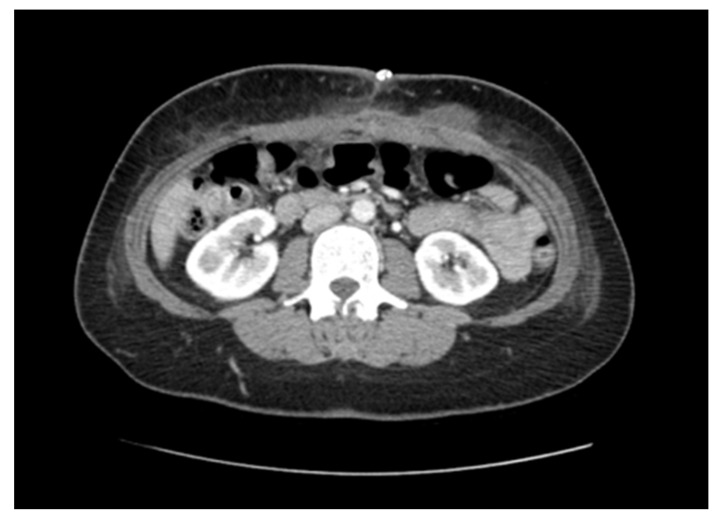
Axial CT imaging 2 weeks post-operatively indicating successful reduction in the hernia contents and repair of the abdominal wall defect. Post-surgical changes in keeping with the expected level at this time period are evident in the abdominal wall. A surgical clip is visible superficially overlying the wound.
